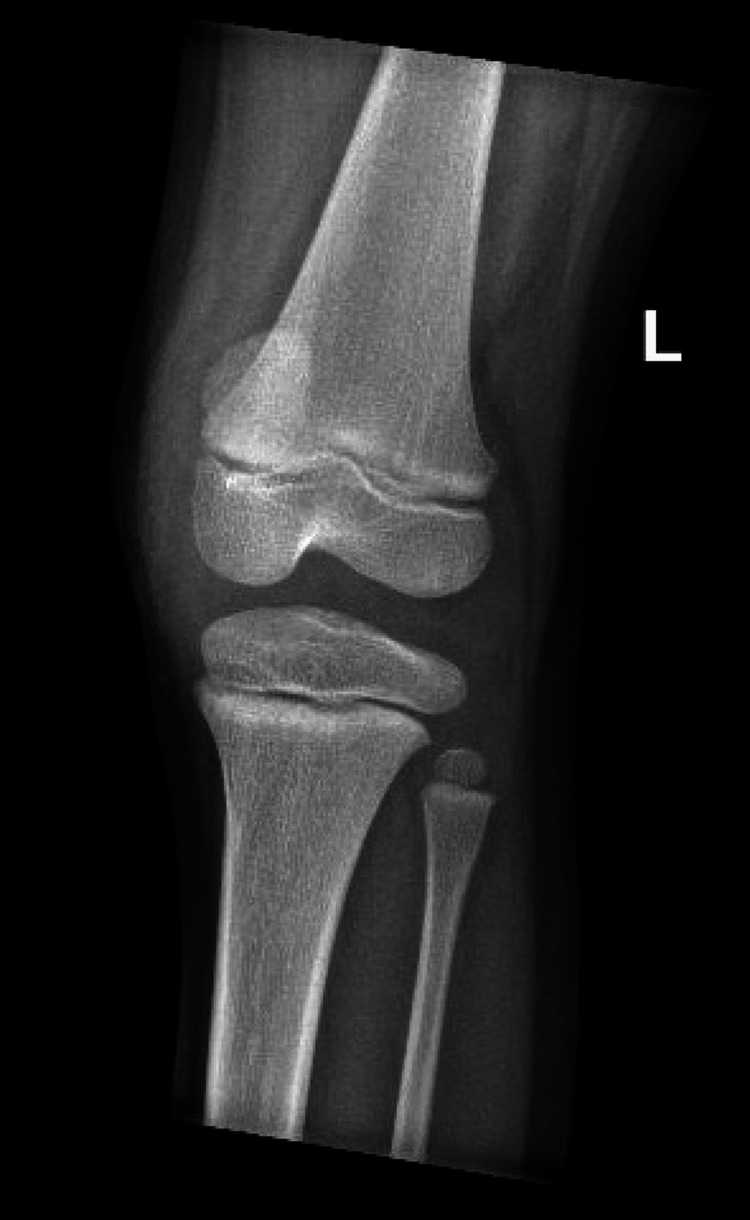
Posterior x-ray view of the left knee.
